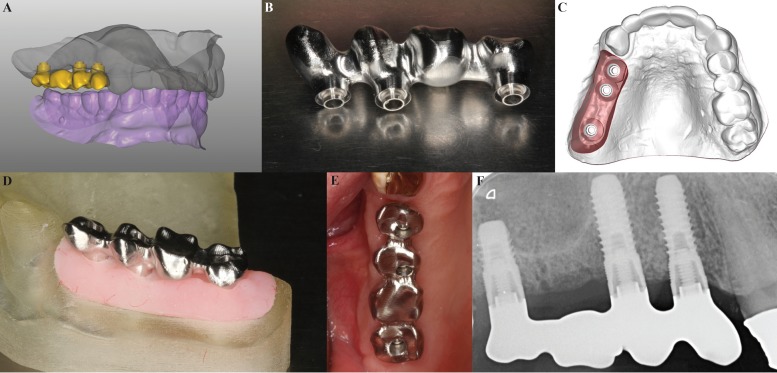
A) Upper and lower plaster models and design of the prosthetic structure; B) Machined metal structure in Cr-Co; C) Digital working model; D) Sterolithography working model with false gums; E) Checking the metal structure in the mouth; F) Periapical radiograph during the Sheffield test.
